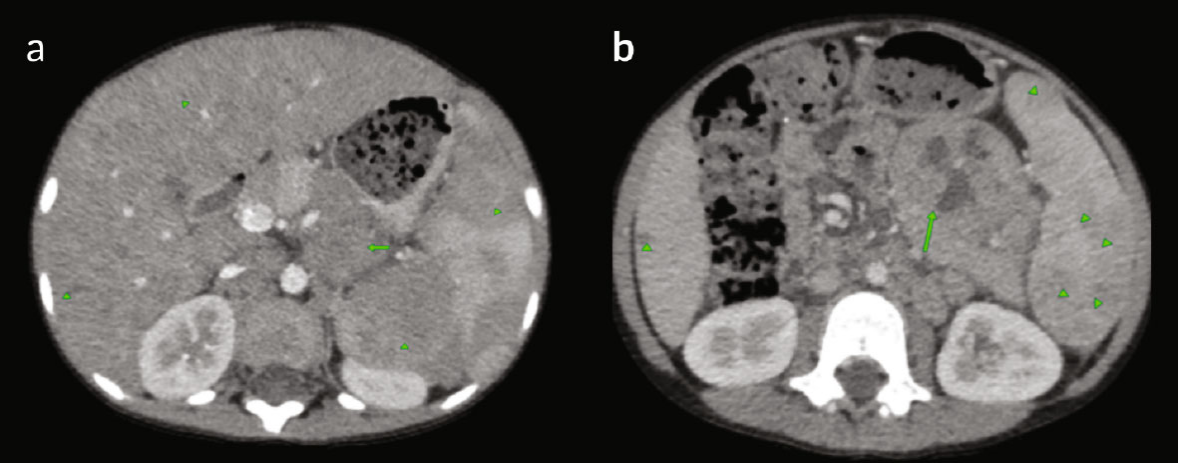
Prechemotherapy postcontrast axial CT images. (a) Diffuse hepatosplenic parenchymal lesions (arrowhead) and a 4 × 5 cm homogeneously hypodense preaortic lesion. (b) A 5 × 7.5 cm mesenteric heterogeneously enhancing mass with central necrosis.
